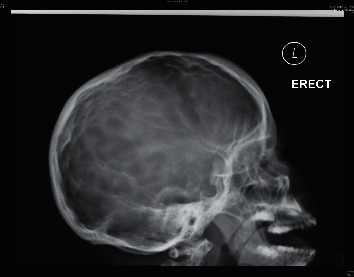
Skull X-ray showing copper beaten skull appearance.
